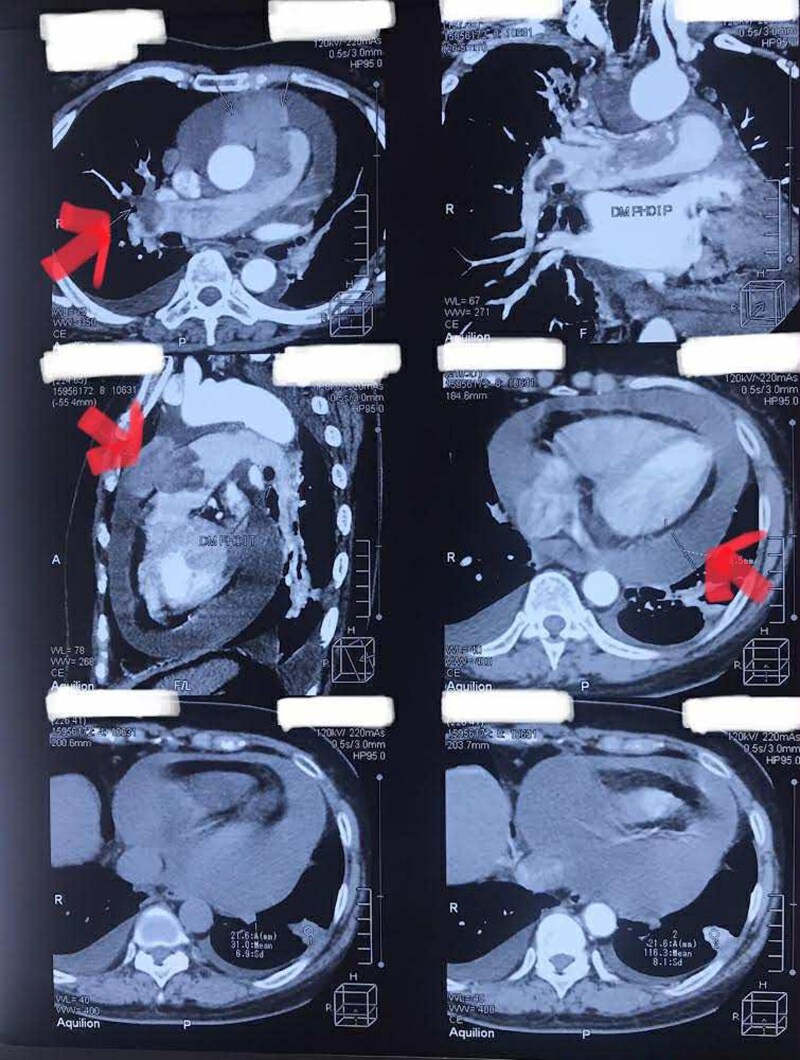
Contrast-enhanced chest computed tomography demonstrating a pericardial mass invading the pulmonary artery, with an associated intraluminal thrombus.
